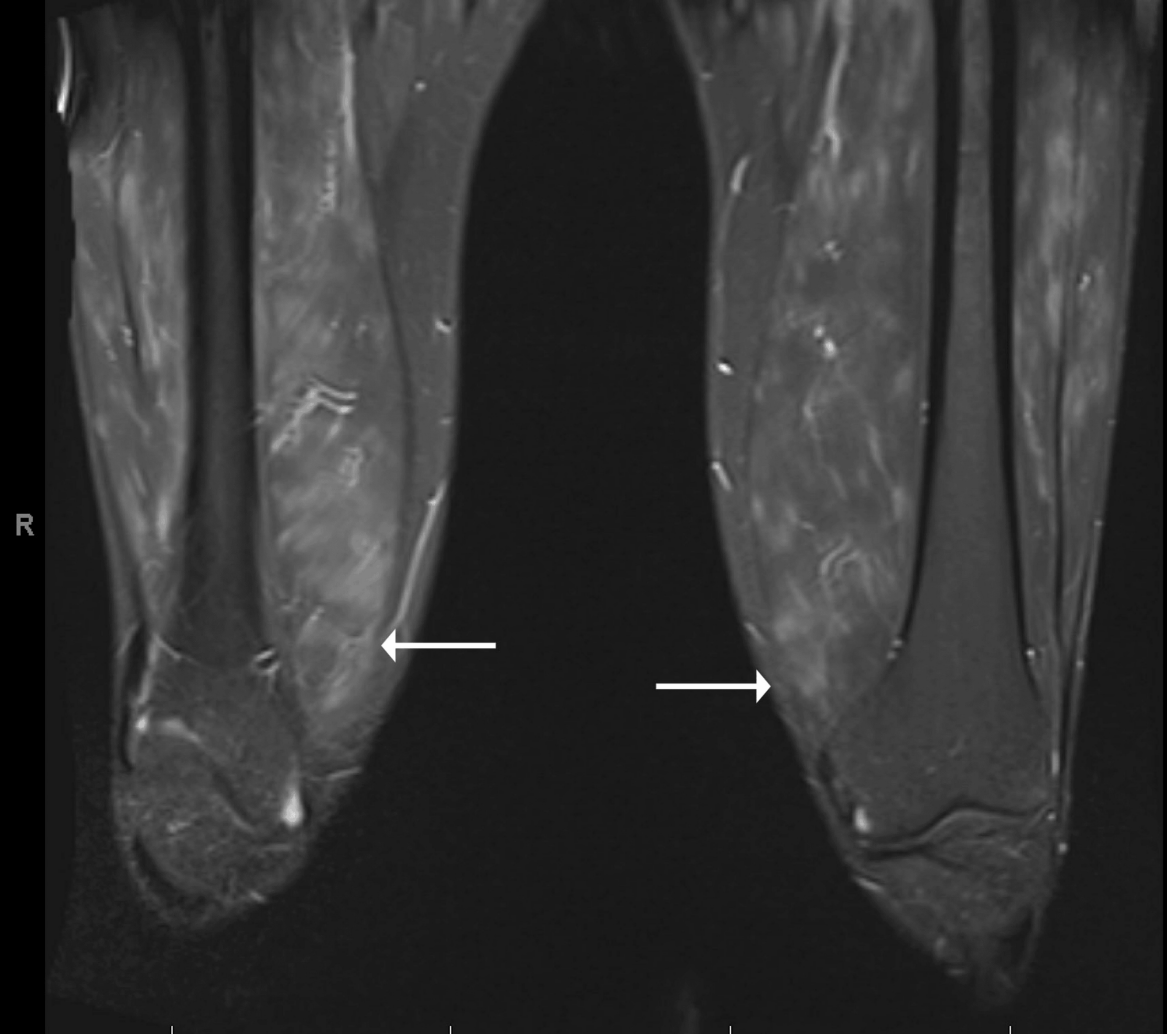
STIR sequence MRI of bilateral thighs shows diffuse feathery muscle edema and enhancement (arrows). STIR, short TI inversion recovery
